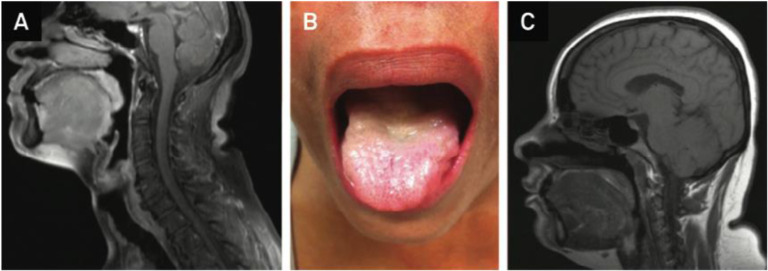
Sagittal T1-weighted brain MRI discloses abnormal diffuse hyperintensity of the tongue musculature (A), correspondent to severe atrophic tongue (B). Normal tongue MRI feature is showed [45].
